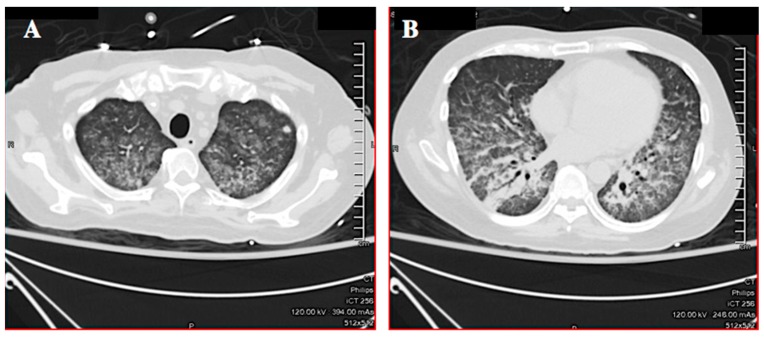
Case 1: CT scan of the chest showing diffuse ground glass airspace opacities, interlobular septal thickening (A), and a right middle lobe cavitary lesion (B). The airspace opacities suggested diffuse alveolar hemorrhage.
